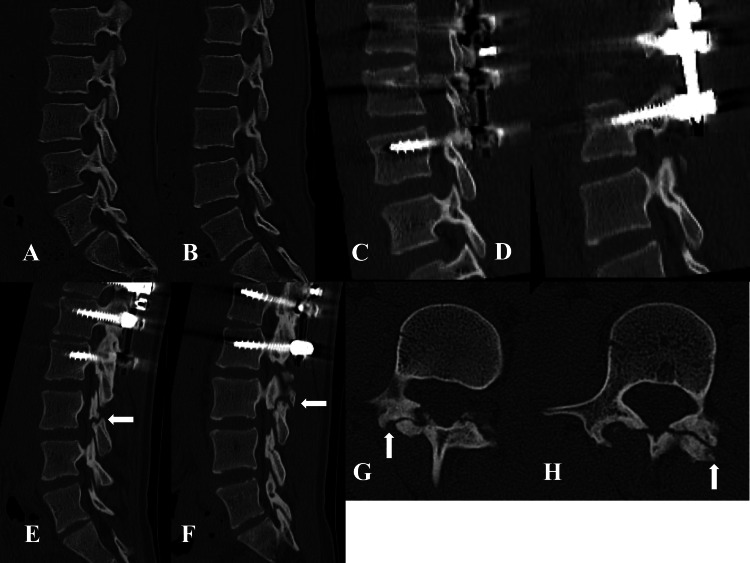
Case 2. Pre- and postoperative sagittal and axial CT images for the PI of L3. Preoperative and one week after surgery CT scans show no abnormalities in the PI of L3 (A: preoperative right PI, B: preoperative left PI, C: right PI one week after surgery, and D: left PI one week after surgery). At a regular check-up, 50 months postoperatively, bilateral L3 spondylolysis was observed (E: sagittal view of right PI, F: sagittal view of left PI, G: axial view of right PI, and H: axial view of left PI). PI: pars interarticularis
